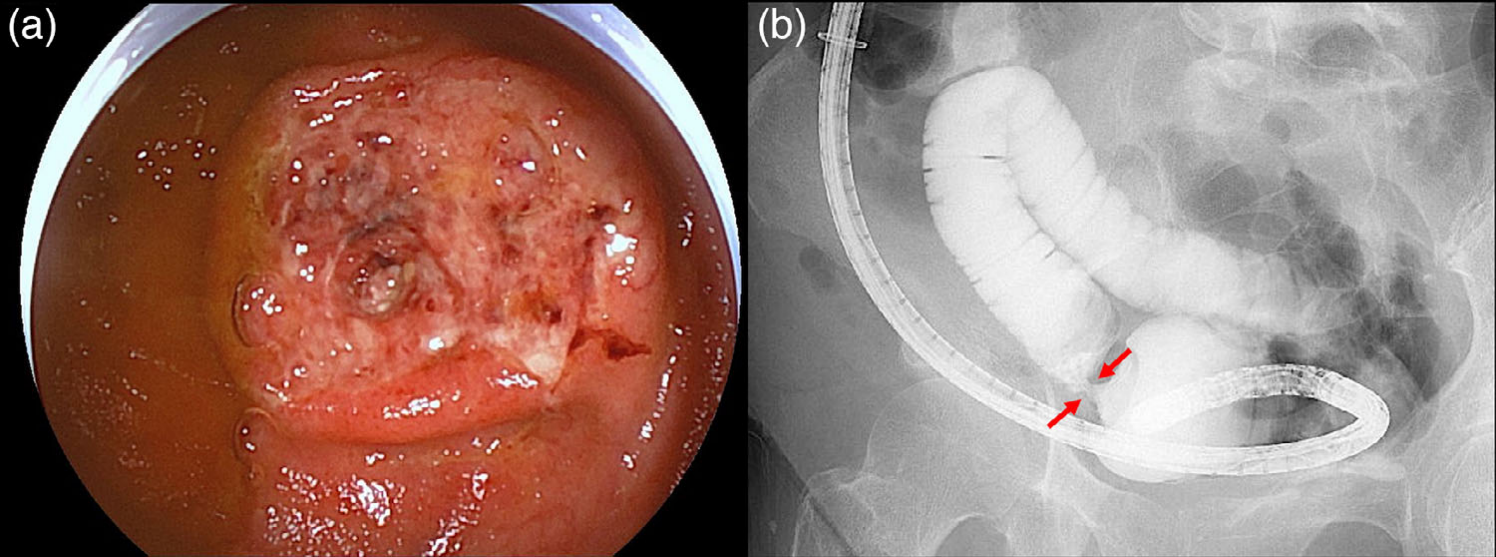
Balloon‐assisted enteroscopy showing an annular ulcer with luminal narrowing in the jejunum (a). Small bowel radiography through the enteroscopy showing an annular stenosis (b, arrows).
